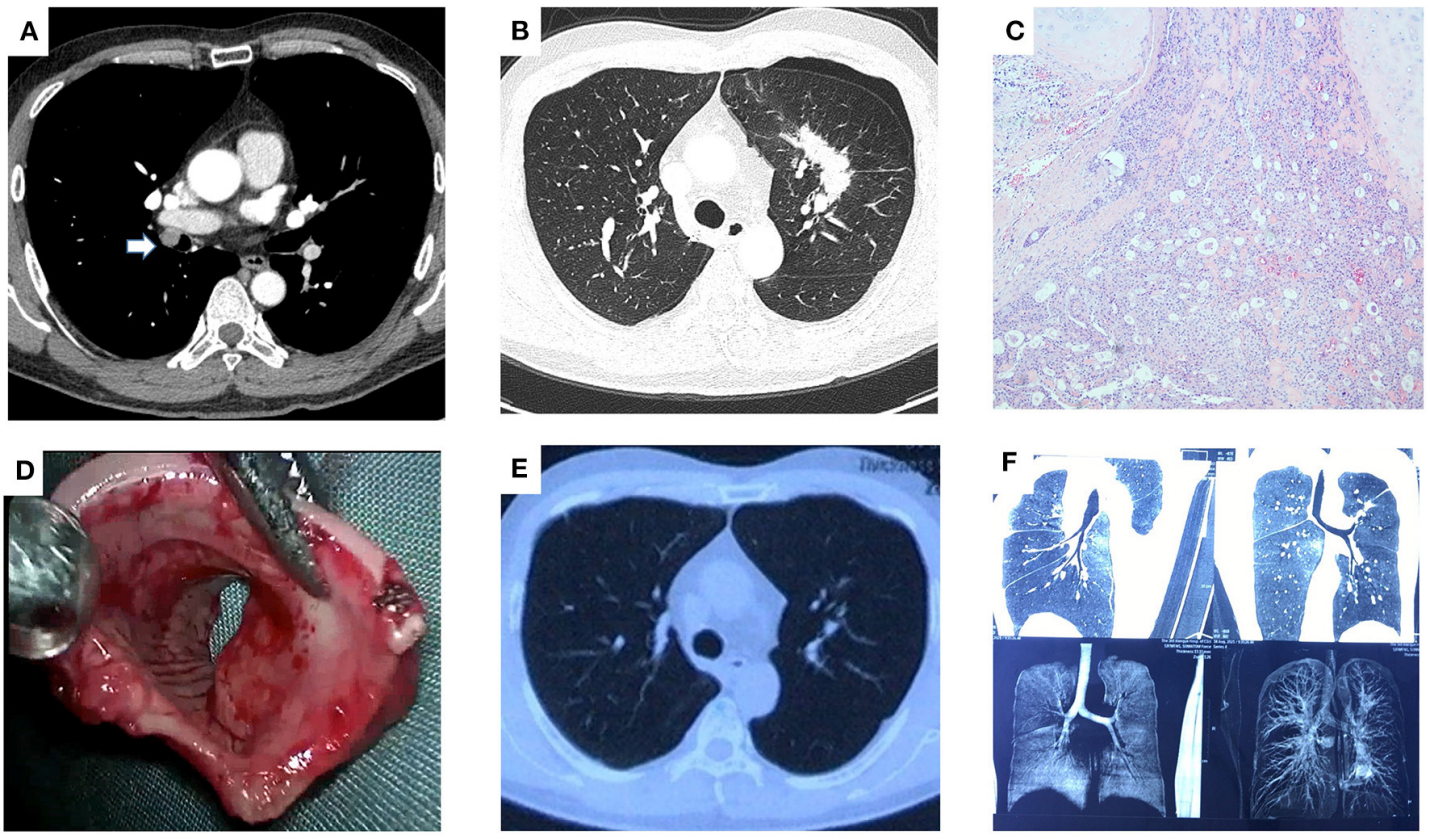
Uniportal VATS secondary carinal resection and reconstruction for tracheobronchial mucoepidermoid carcinoma. Contrast-enhanced CT of the chest showed a 1.5 × 1.2 cm endobronchial nodule of the right bronchus intermedius (see arrow, A) and a 5.1 × 3.1 cm patchy lesion centrally located at the left upper lobe (B). (C) Postoperative pathology confirmed the diagnosis of the well-differentiated mucinous epidermoid carcinoma. (D) Gross appearance of the endobronchial mass. (E) Contrast-enhanced CT of the chest at the 6-month postoperative follow-up showed resolution of tuberculosis lesion in the left upper lobe. (F) CT bronchography at the 6-month postoperative follow-up showed no bronchial stenosis of the right lung.
